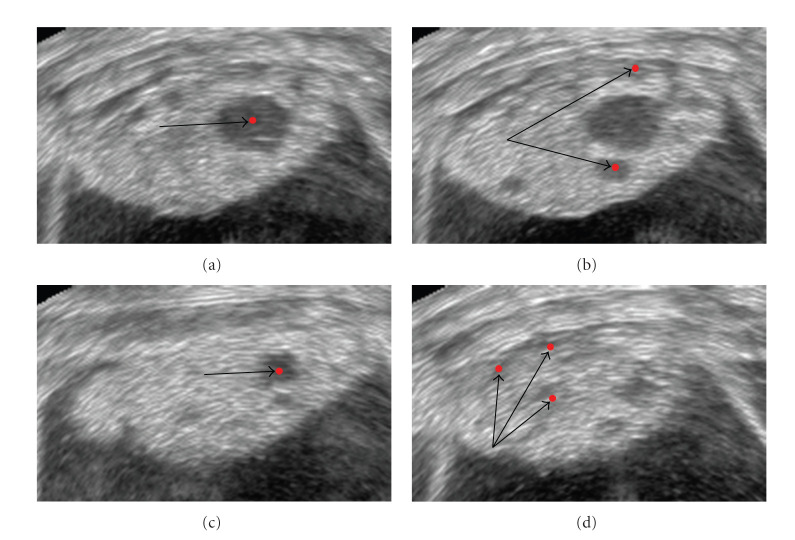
Follicle detection. 2D slices of the 3D ultrasound image smoothed data set corresponding to one of the patients. Lighter areas display the ovary; dark circular blobs are the follicles. Red dots indicate retrieved local minima in 3D at scale *s* = 9 voxels.
